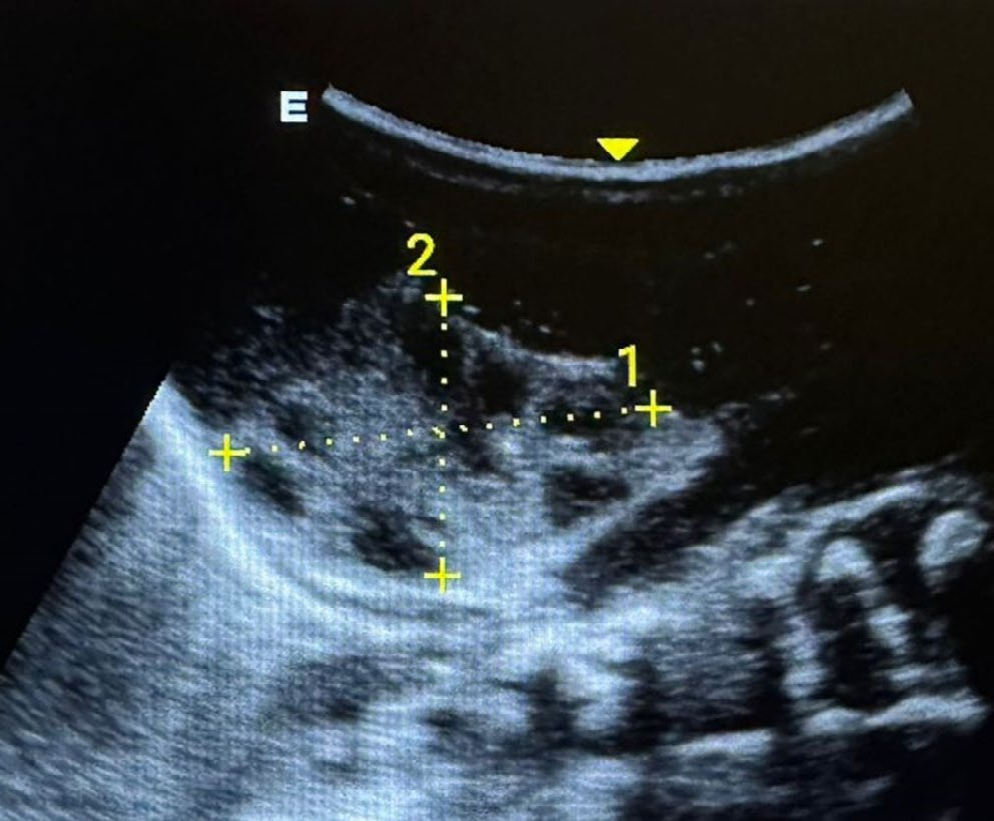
Ultrasonography images 1 day after delivery showing multiple cysts in the kidney.
